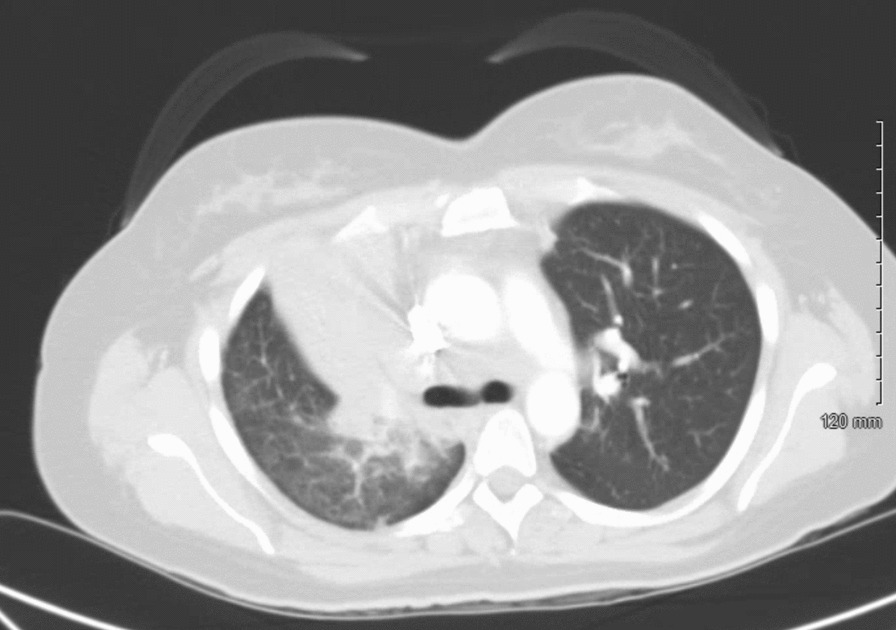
Axial section of a CT of the chest with intravenous contrast demonstrating right upper lobe consolidation with collapse
